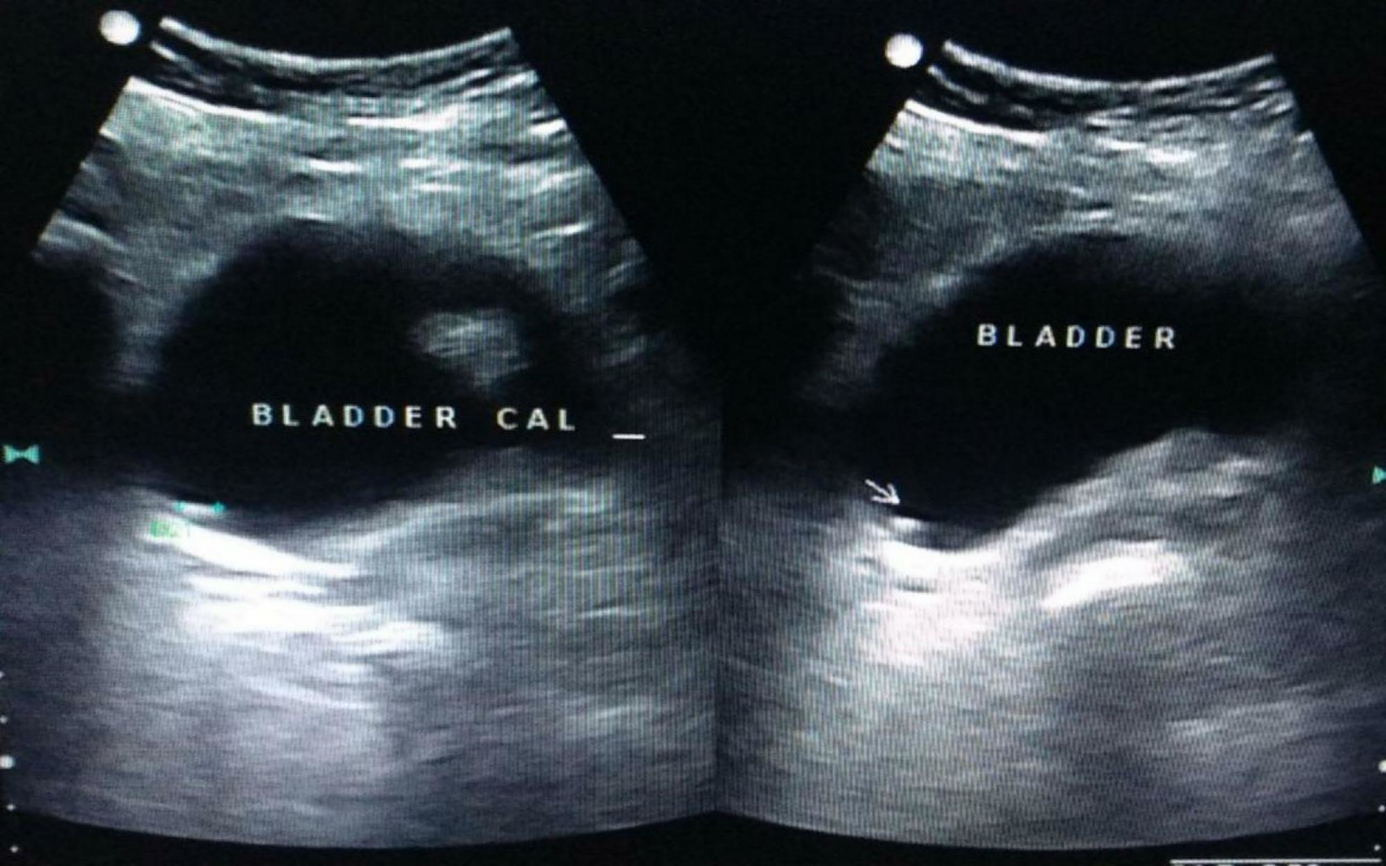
Ultrasonogram KUB (kidney urinary bladder) - Revealed 1.2 cm right upper pole renal calculus with 1.7 cm vesical calculus (arrow).
